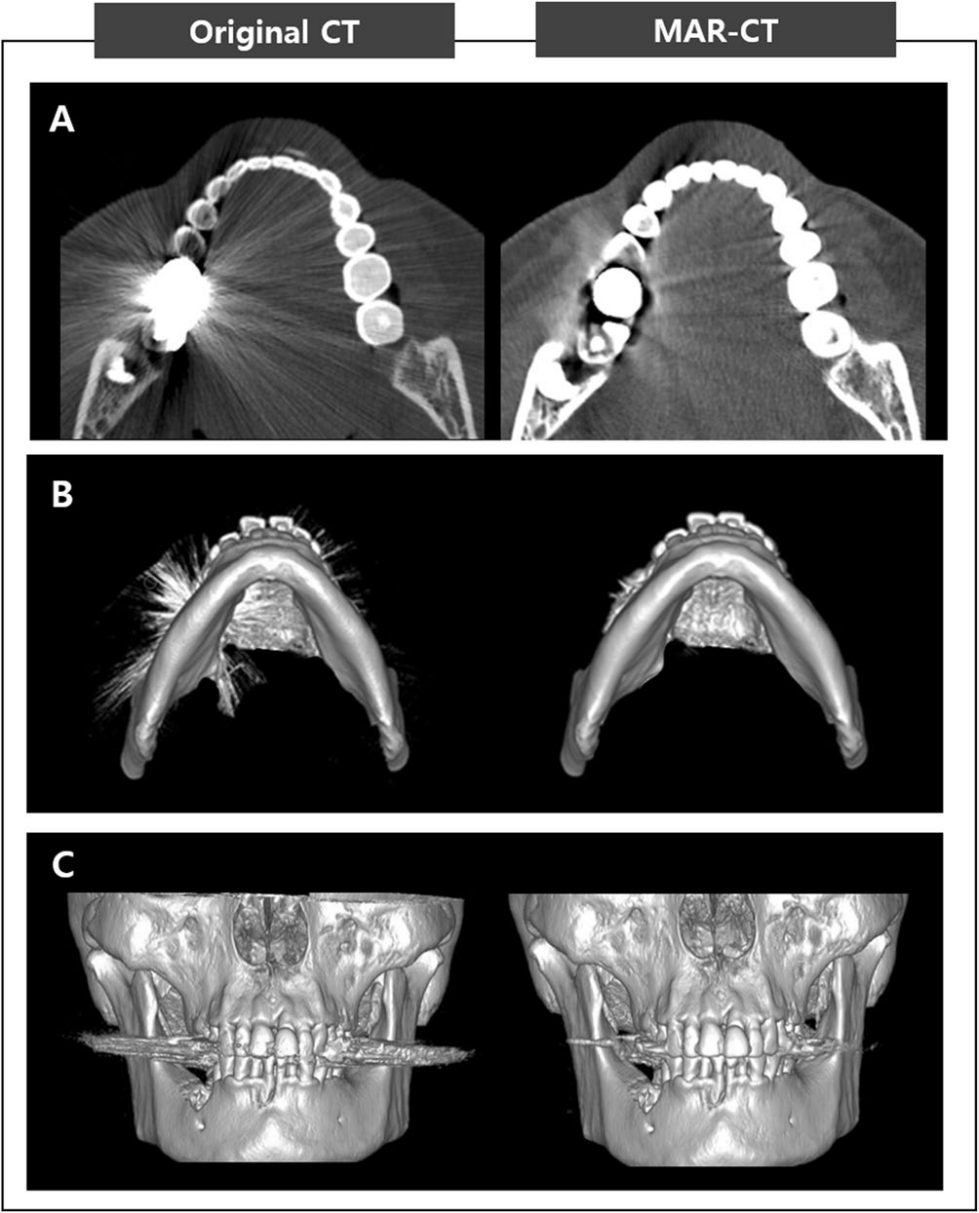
Original CT and MAR-applied CT. (A) Axial view, (B) volume rendering image (bottom view), (C) volume rendering image (frontal view). Note the significant reduction of metal artifacts in the MAR-CT image compared to the original CT. *MAR* metal artifact reduction.
